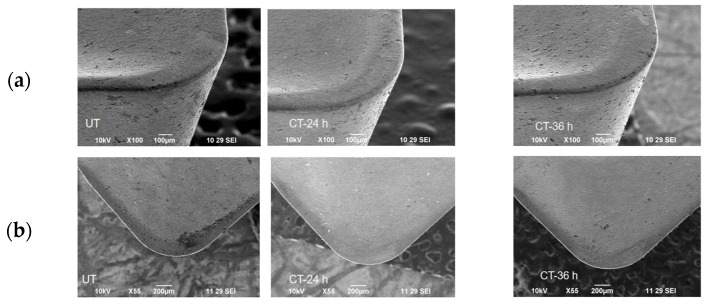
SEM image cutting insert at spindle speed of 3100 rpm, feed rate of 0.1 mm/tooth of UT, 24-h CT and 36-h CT, (a) flank face and (b) rake face.
